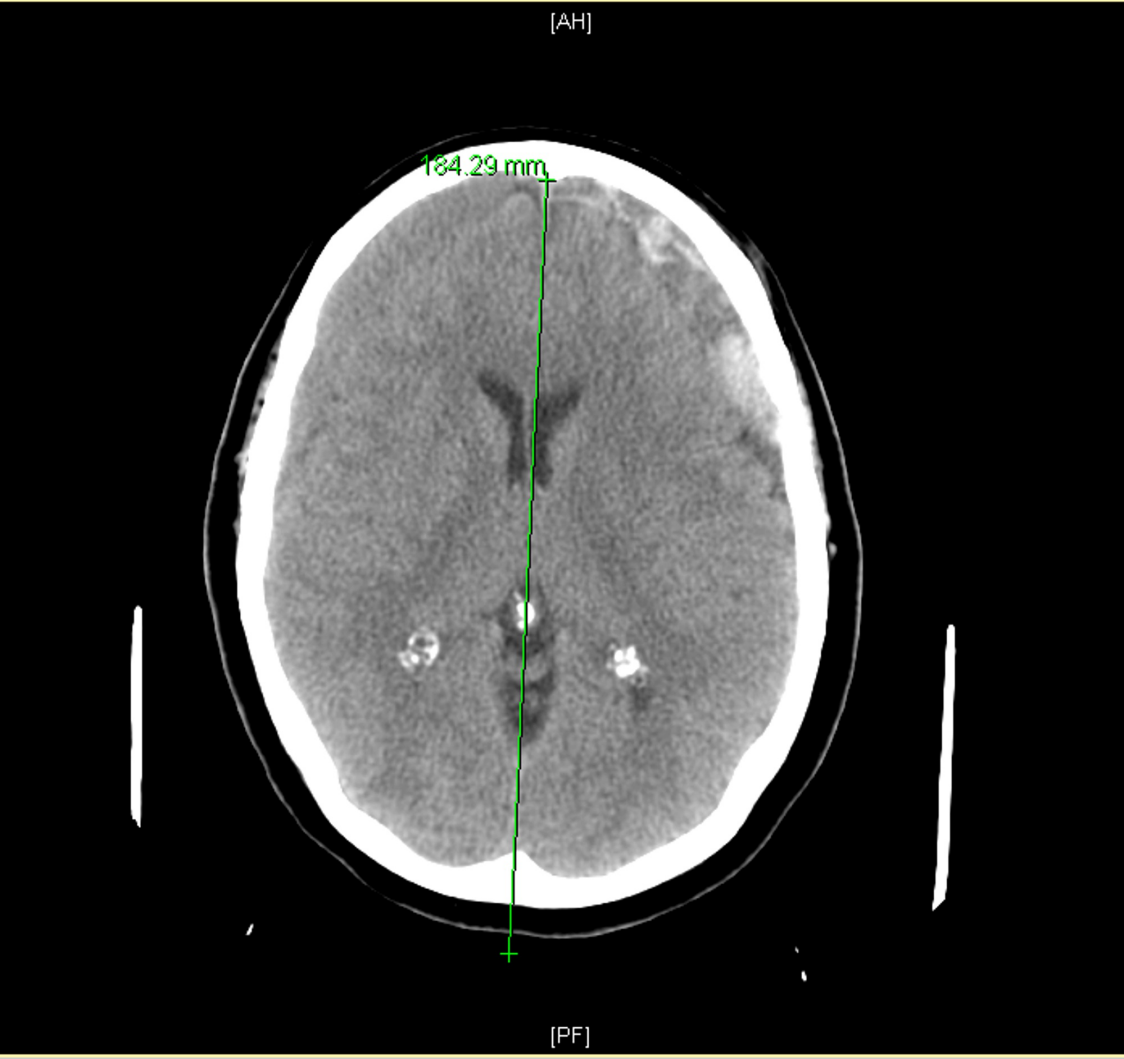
Preoperative head CT scan reveals bilateral SDH with mass effect. Effacement of the bilateral sulcal gyral pattern is observed CT: Computed tomography; SDH: Subdural hematoma.
